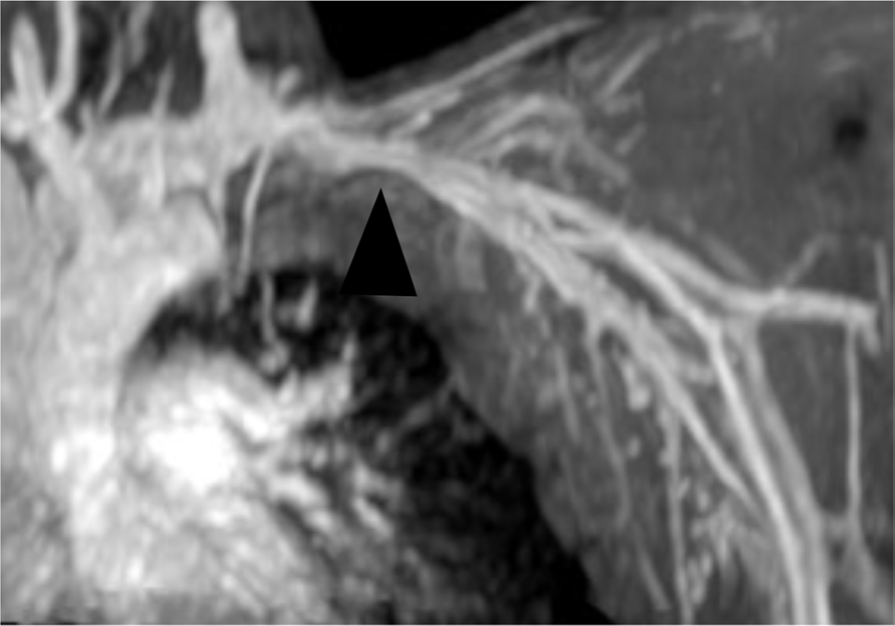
Follow-up magnetic resonance imaging scan conducted at the 3-month post-procedural visit demonstrating regular SV patency (black arrowhead)
